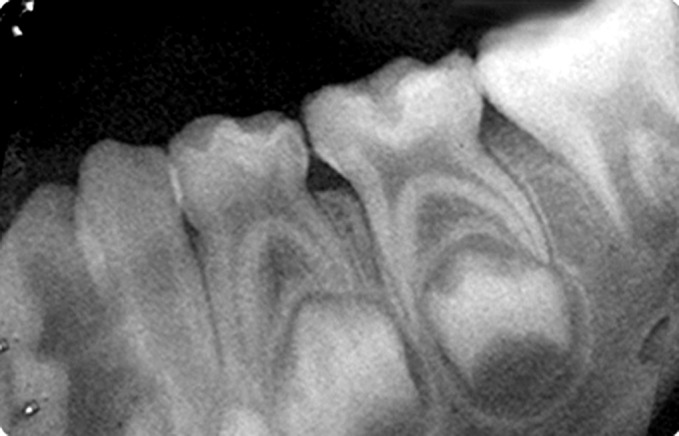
Preoperative IOPA radiograph of glutaraldehyde
